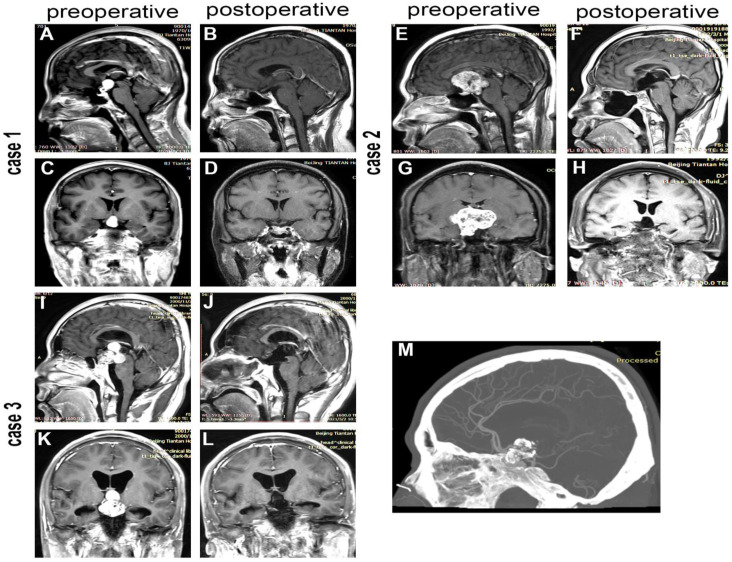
The application of EETS in resecting craniopharyngioma. Case 1 patient with preoperative hypothalamic involvement had improved hypothalamic involvement after EETS. Preoperative sagittal (A) and coronal (C) MR T1-weighted images showed tumor invasion of the hypothalamus; postoperative sagittal (B) and coronal (D) MR T1-weighted images showed the integrated hypothalamus. Case 2 patients with giant craniopharyngioma underwent gross total resection (GTR) using EETS. Preoperative sagittal (E) and coronal (G) MR T1-weighted images showed craniopharyngioma of giant size; postoperative sagittal (F) and coronal (H) MR T1-weighted images showed the GTR. Case 3 patients with calcified craniopharyngiomas underwent GTR using EETS. Preoperative sagittal (I), coronal (K) MR T1-weighted images, and preoperative sagittal (M) computed tomography images showed the craniopharyngioma possessed calcification; postoperative sagittal (J) and coronal (L) MR T1-weighted images showed the GTR.
